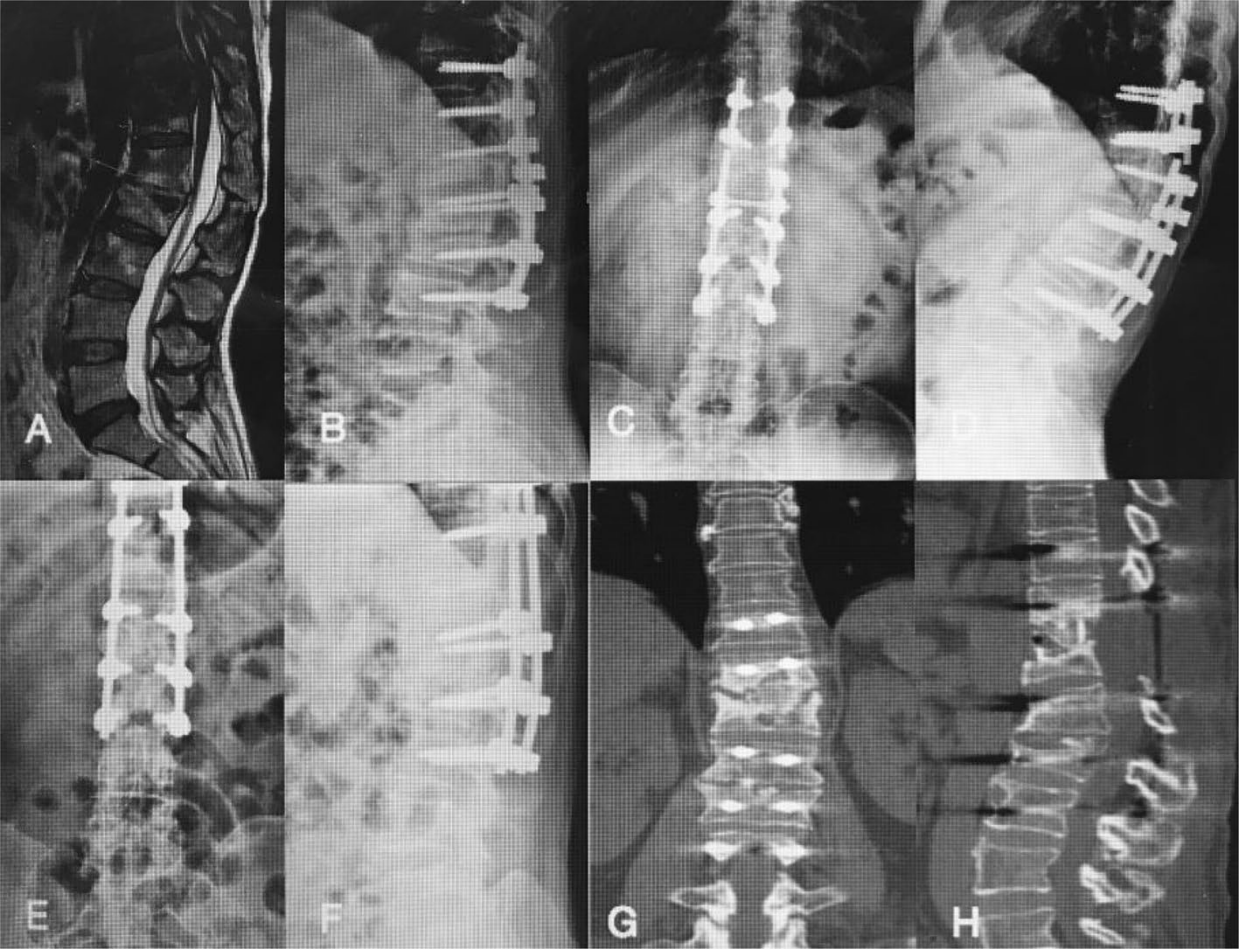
A 51-year-old woman with thoracic spinal tuberculosis (T11–12) underwent posterior debridement and decompression combined with instrumentation. (A,B) Preoperative computed tomography (CT) shows bone destruction of the T11–12 vertebrae and compression of the spinal cord. (C,D) The 2-year postoperative X-ray and CT demonstrate the bone was unhealed, and a rod fracture was observed. (E–H) The revision surgery was performed with an iliac graft. X-ray and CT show the good location of the bone graft.
